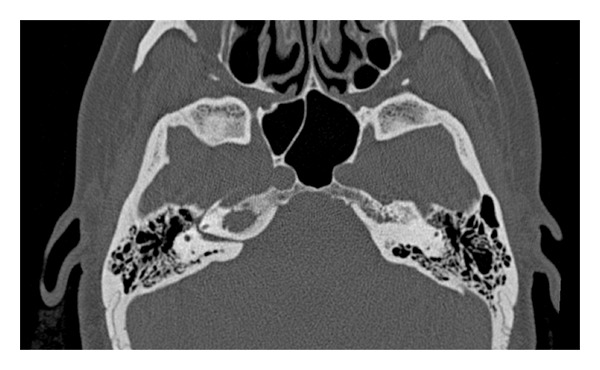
Axial CT scan showes an osteolytic lesion involving the right petrous apex.
